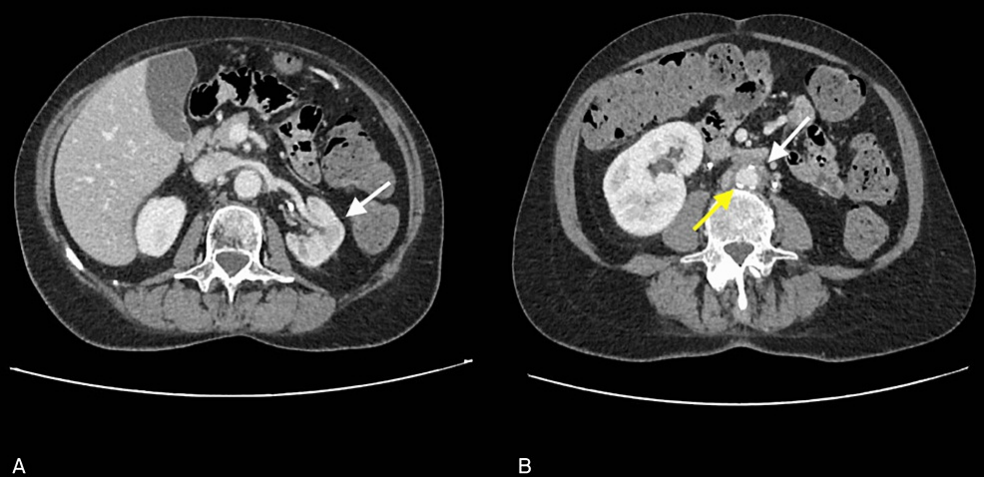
CT abdomen with contrast enhancement: resolution of obstructive nephropathy (arrow in A), thickening of periaortic and pericaval adipose tissue (white arrow in B), aortic atherosclerosis (yellow arrow in B). Bilateral pyelovesical stents in place; no dilatation of the calyceal-pelvic cavities observed. The left kidney is of reduced size with thinned parenchymal thickness (A). The right kidney is enlarged, albeit to a lesser extent compared to the previous reference. Thickening of adipose tissue in the para-aortic and paracaval regions is observed (B).
